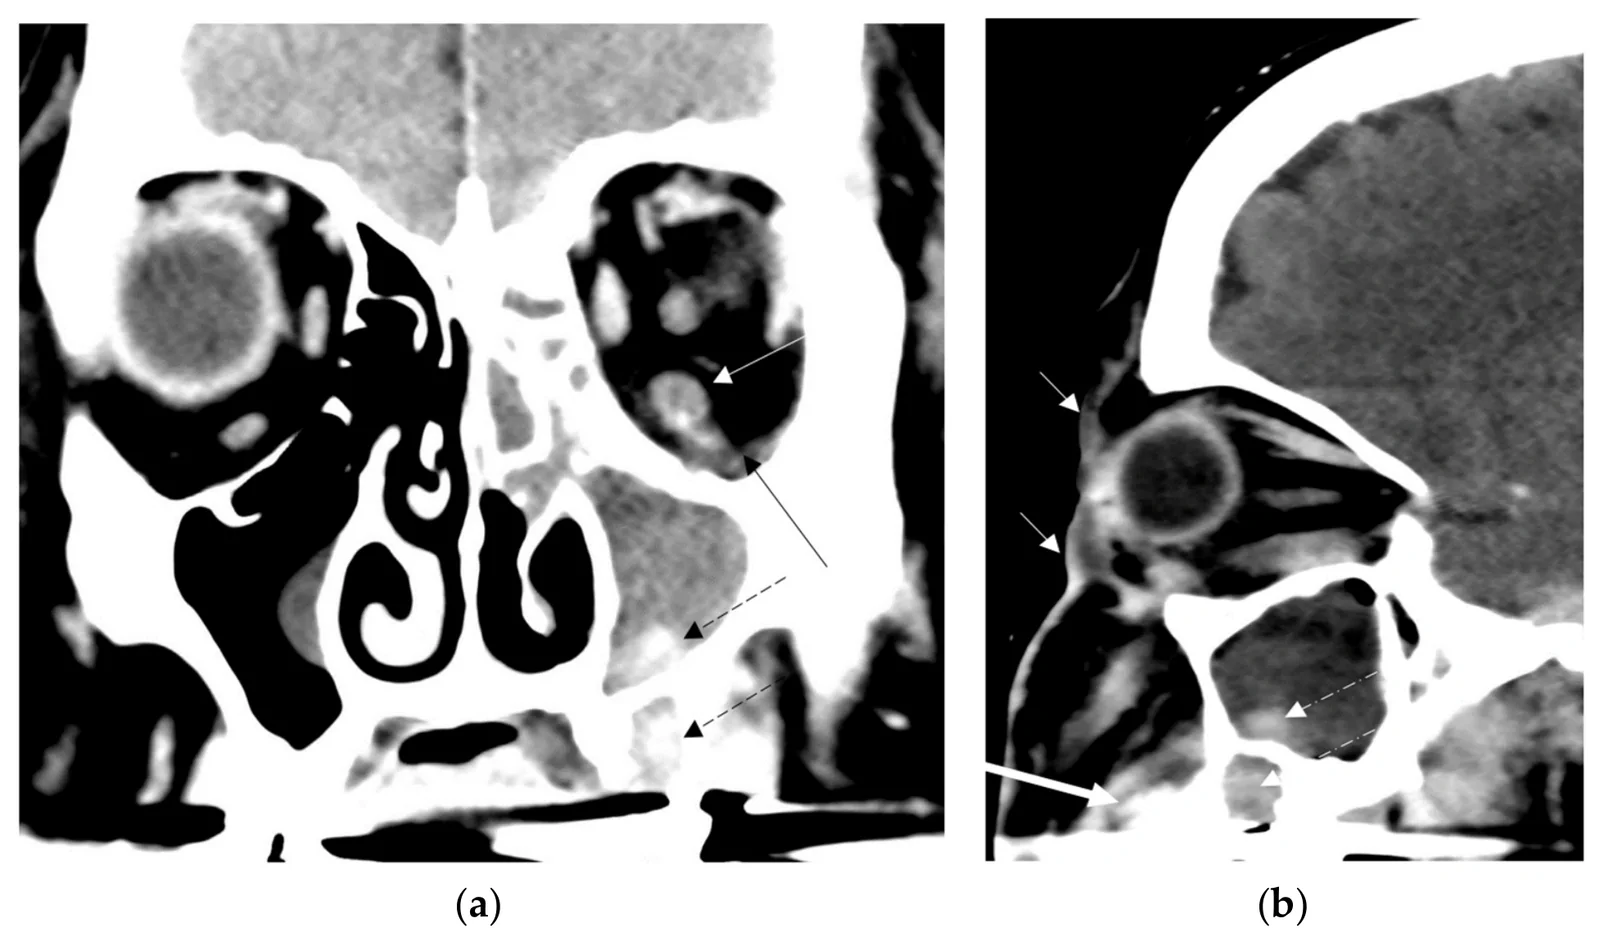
(a) Coronal reformat of CT head post-contrast demonstrating diffuse fat stranding in the left post-septal orbital space (black arrow) with slight enlargement of the extraocular muscles (white arrow). There is diffuse opacification of the left sides’ sinuses—in the floor of the left maxillary sinus with underlying odontogenic cyst (black dashed arrows). (b) Sagittal reformat of CT head post-contrast on the left side demonstrating diffuse fat stranding in the left pre-septal periorbital space (white short arrows) with slight enlargement of the extraocular muscles—in the floor of the left maxillary sinus with underlying odontogenic cyst (white dashed arrows) and abscess in the adjacent gingiva (white arrow).
